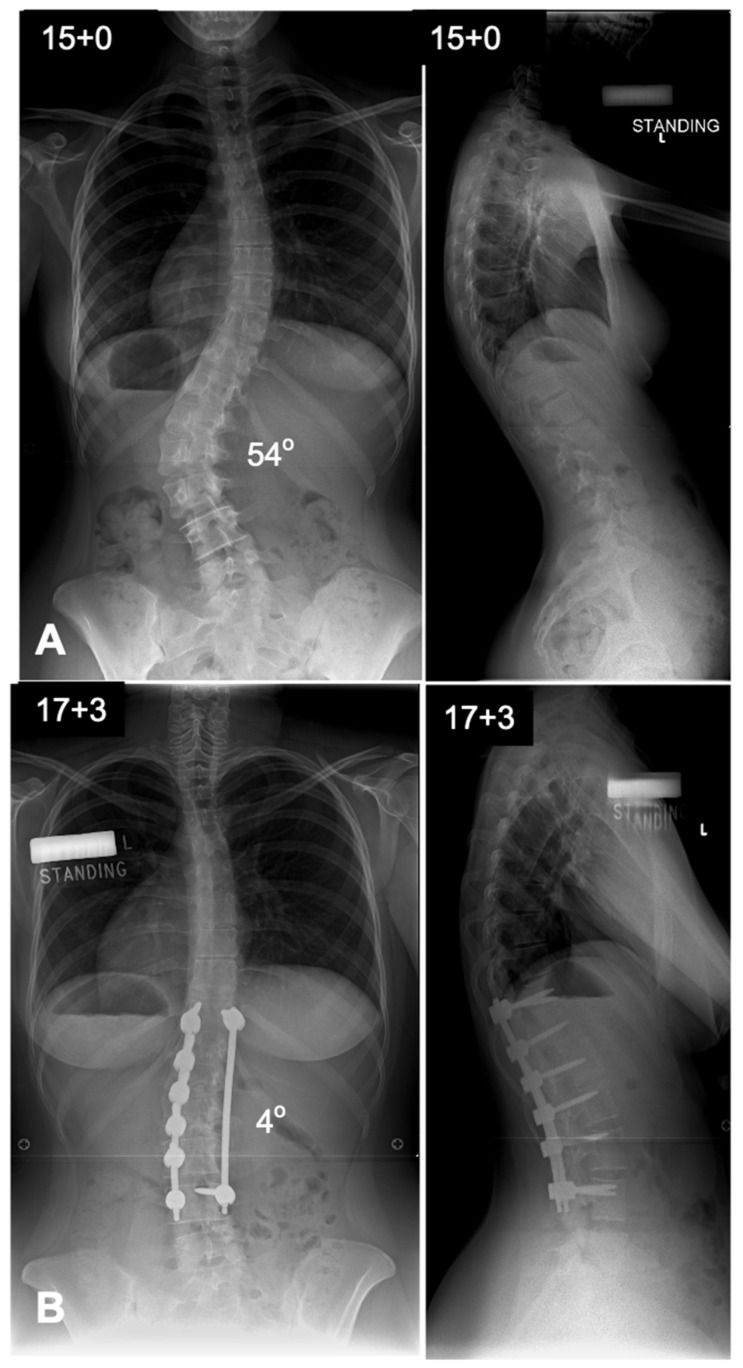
Deformity correction using posterior spinal fusion in a Lenke 5 curve. (A) Preoperative antero-posterior and lateral radiographs showing a thoraco-lumbar scoliosis with a Cobb angle of 54 degrees. (B) Postoperative radiographs showing good deformity correction with coronal and sagittal balance. Correction in posterior spinal fusion in thoraco-lumbar and lumbar curves is achieved from the convex rod.
